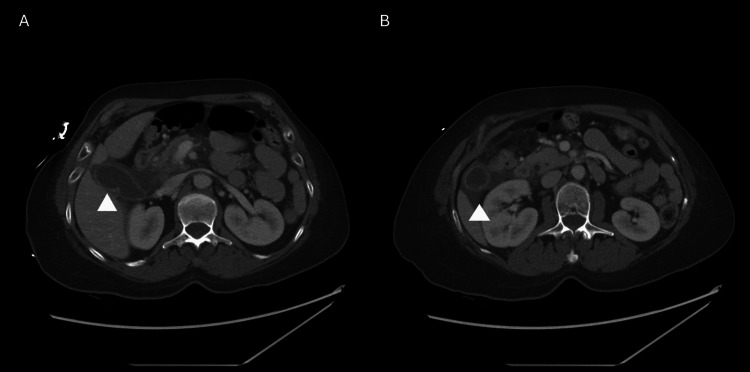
Representative CT images demonstrating (A) gallbladder wall thickening consistent with acute cholecystitis (arrow) and (B) free pericholecystic fluid consistent with perforated cholecystitis
